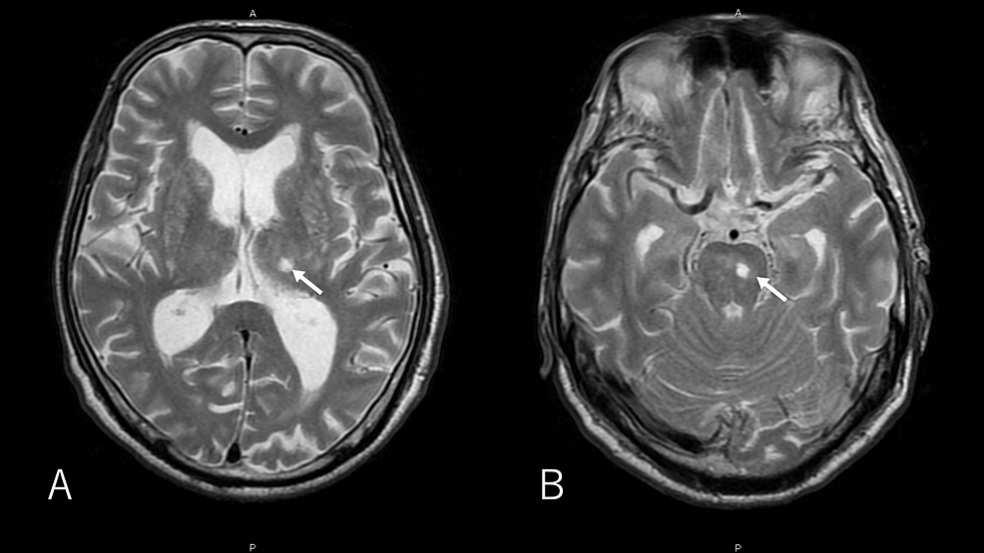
Magnetic Resonance Imaging (T2WI sequence) on the third day of hospitalization showing high signal in the left thalamus (A) and left side of the pons (B).
